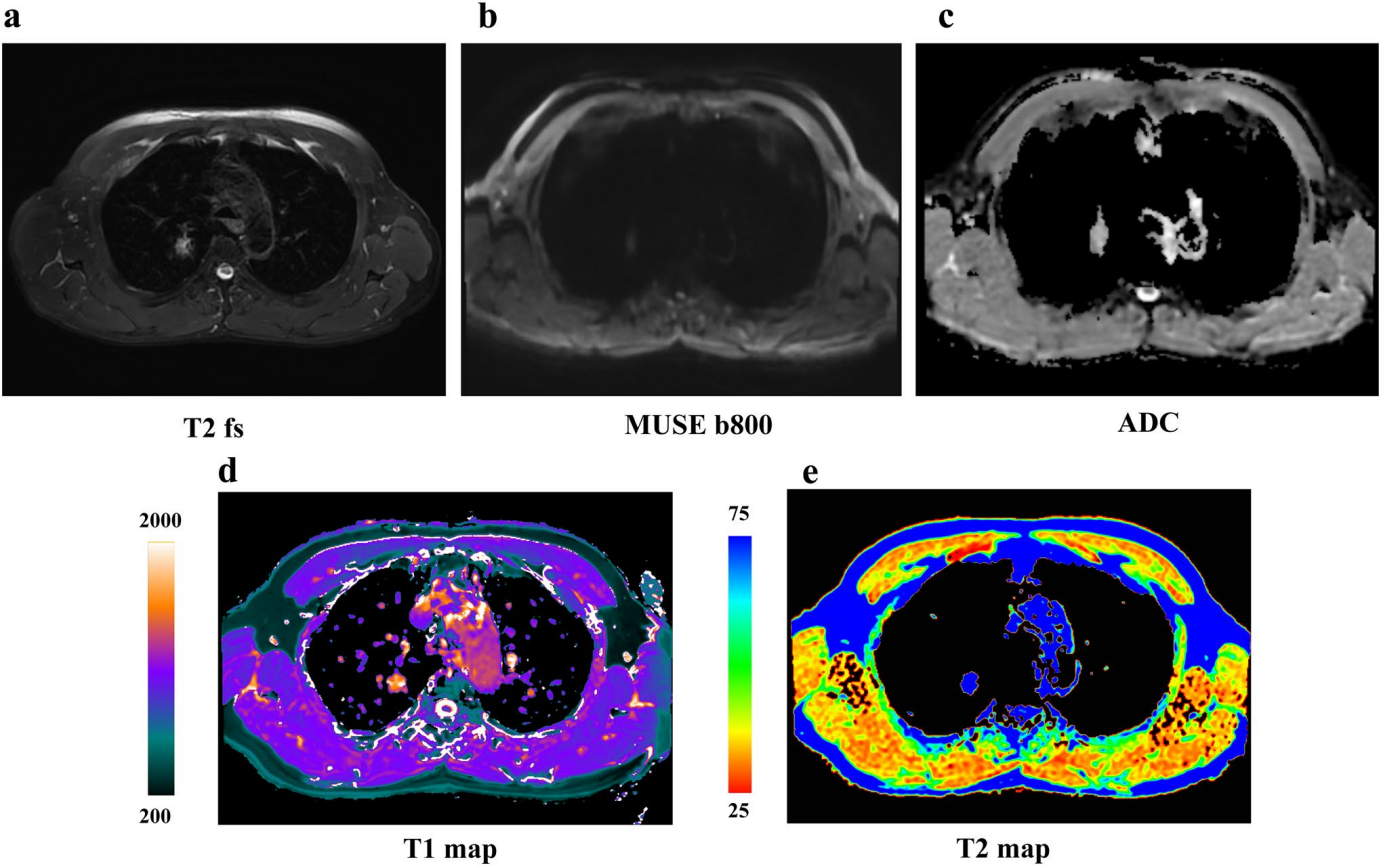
Male, 63 years old, nodule in the upper lobe of the right lung, approximately 13 mm in size, Pathology confirmed inflammatory granuloma. (a) T2WI; (b) MUSE DWI (*b* = 800 s/mm^2^); (c) MUSE ADC; (d) T1 mapping (ms); and (e) T2 mapping (ms).
